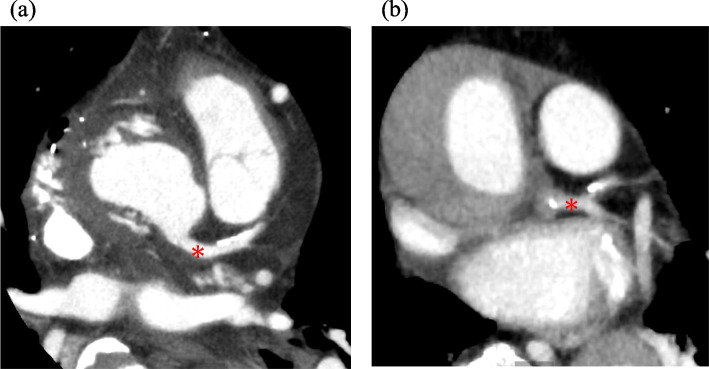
Computed tomography. a Preoperative and b postoperative left main trunk (LMT) (*). The dissection of the LMT has resolved
